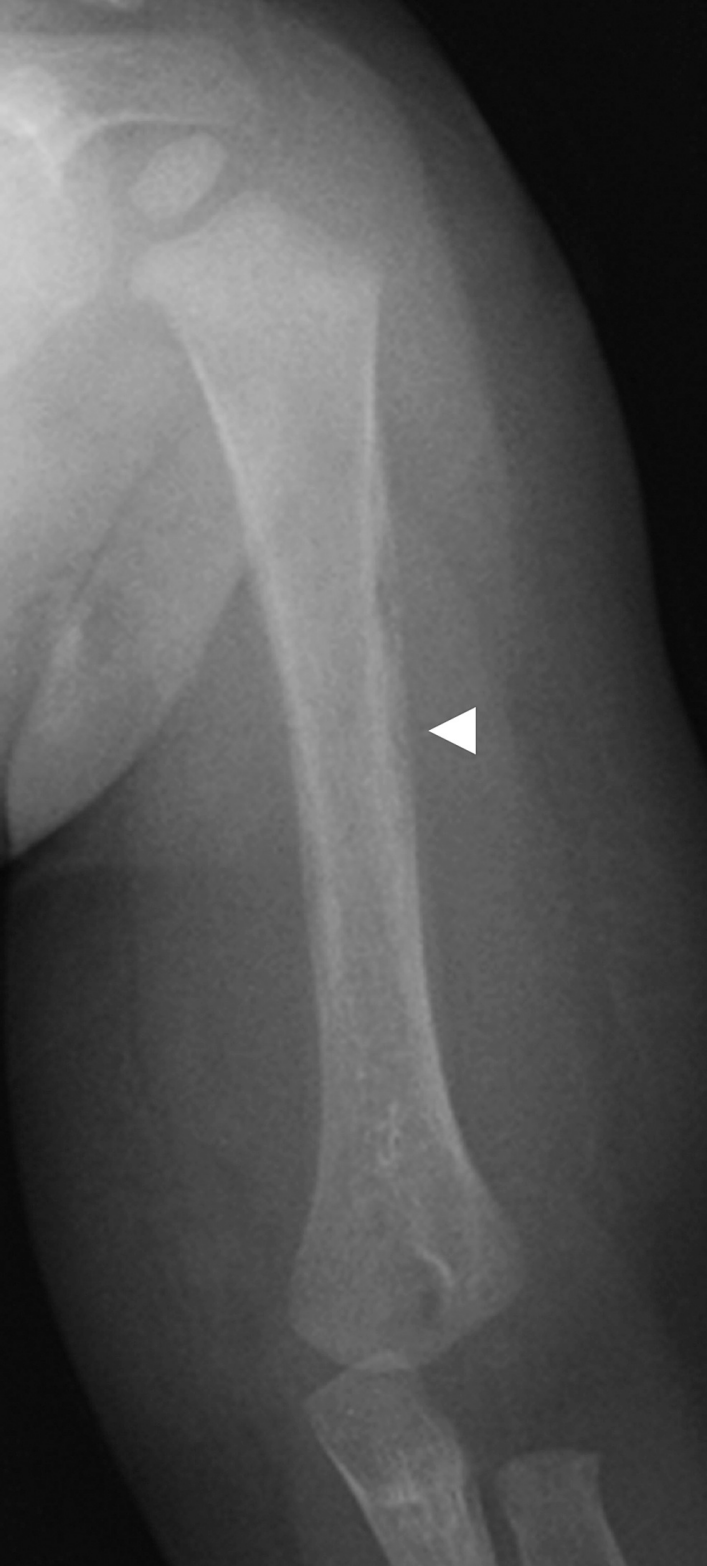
Anteroposterior radiograph of the left humerus taken at the first visit showing a diaphyseal osteolytic bone lesion and a periosteal reaction (arrowhead).
